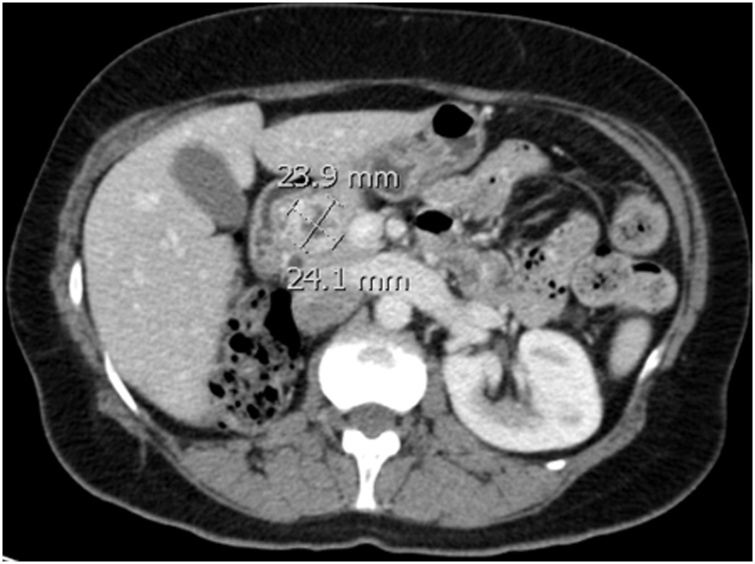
Axial view CT scan of the abdomen showing a metastatic lesion in the head of the pancreas.
